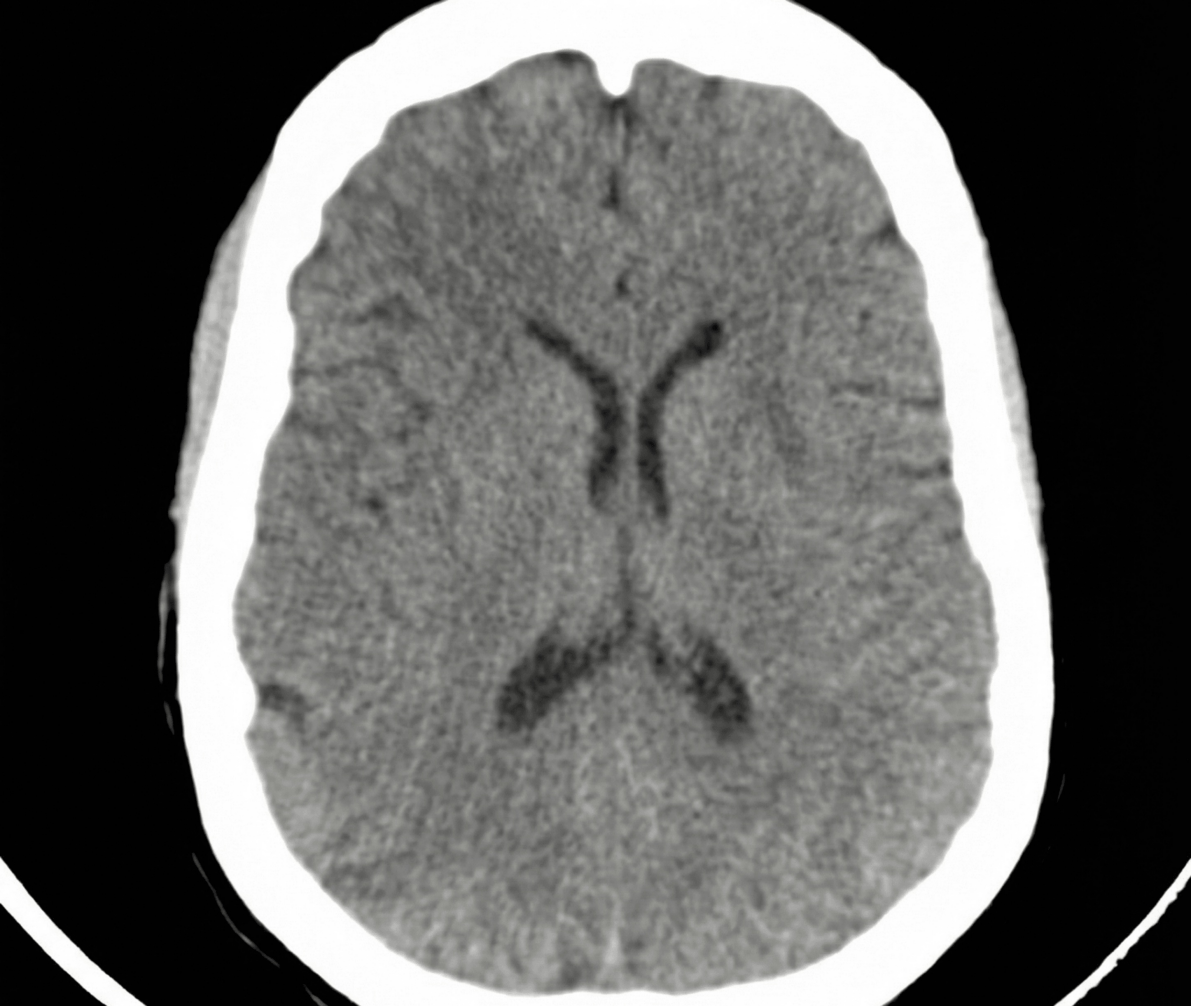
Computed tomography brain indicative of posterior reversible encephalopathy syndrome (PRES)
